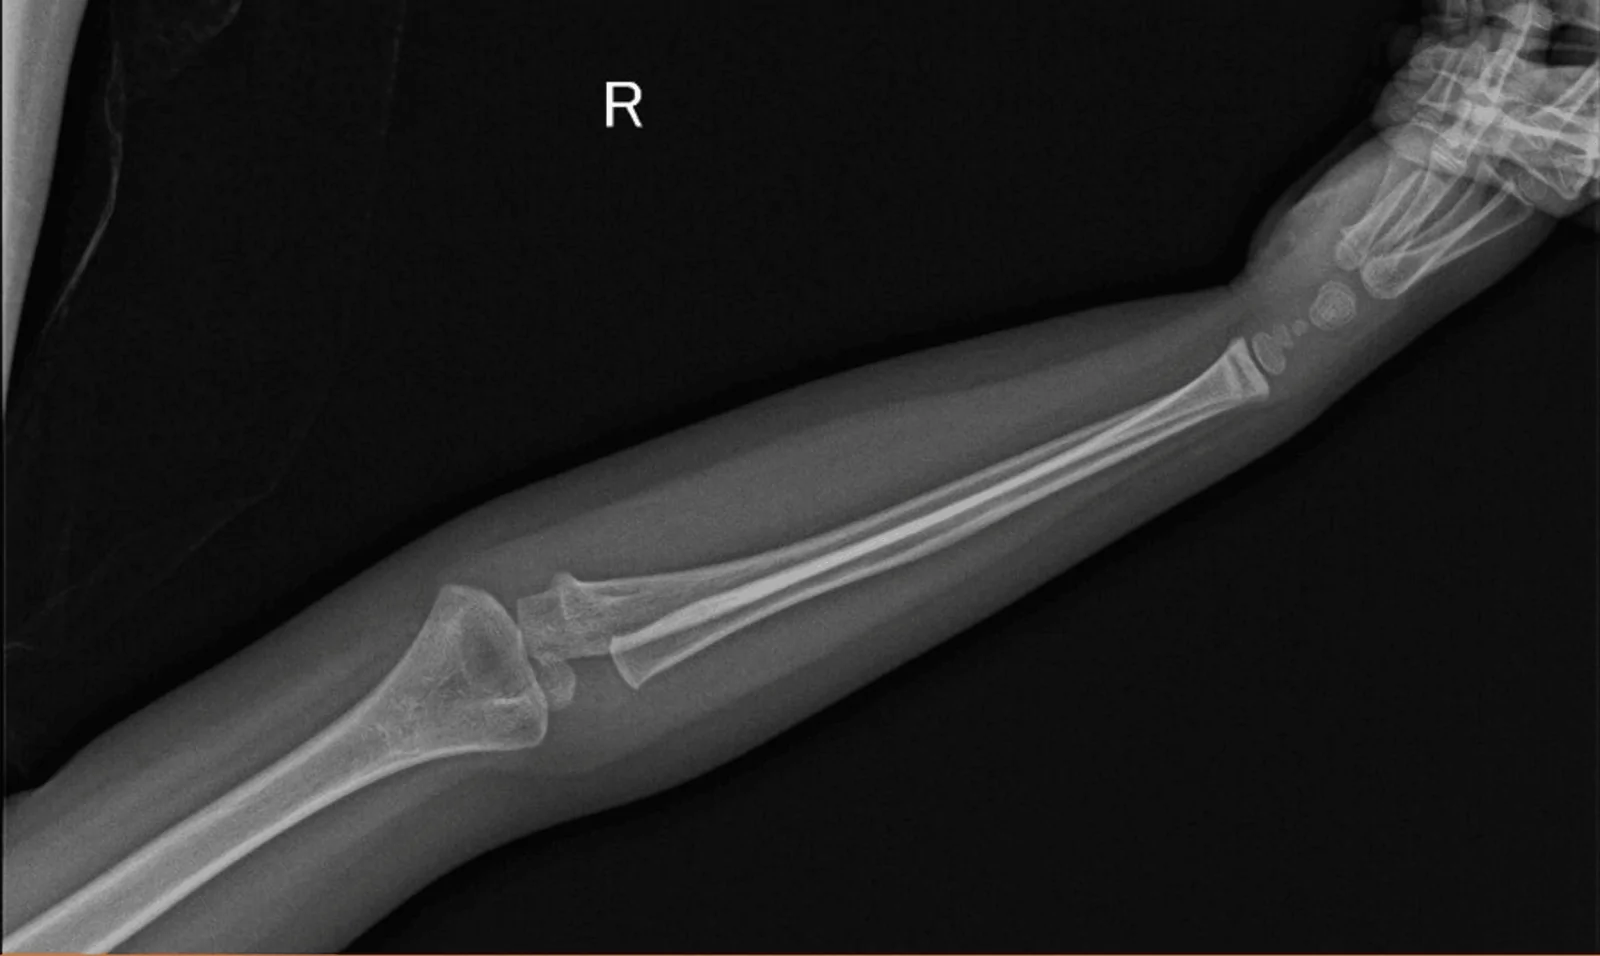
Lateral Radiograph of the Right Elbow No acute fracture or dislocation is identified, with preserved alignment.
